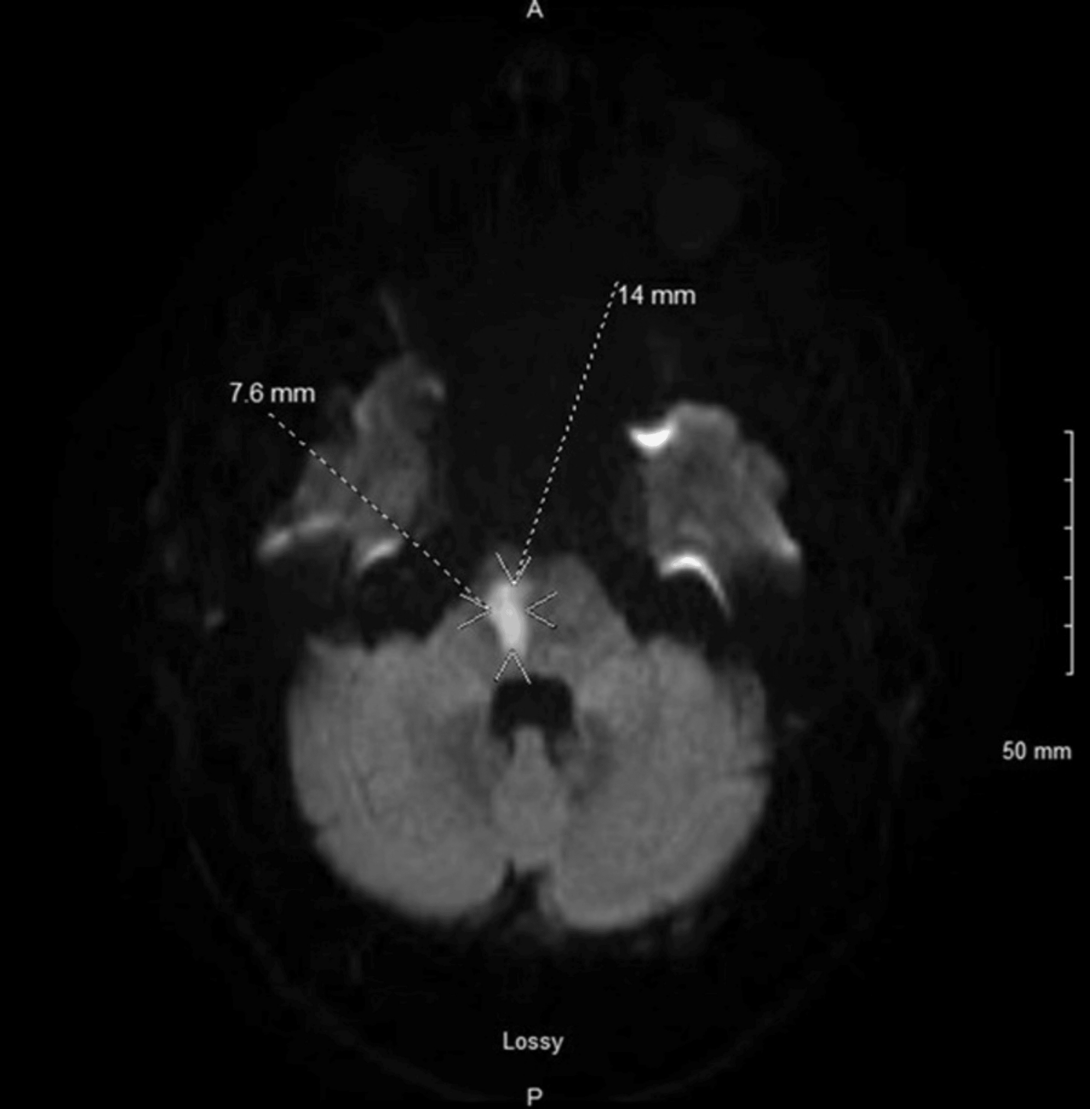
A pontine stroke of 14 mm x 7.6 mm seen on the MRI
